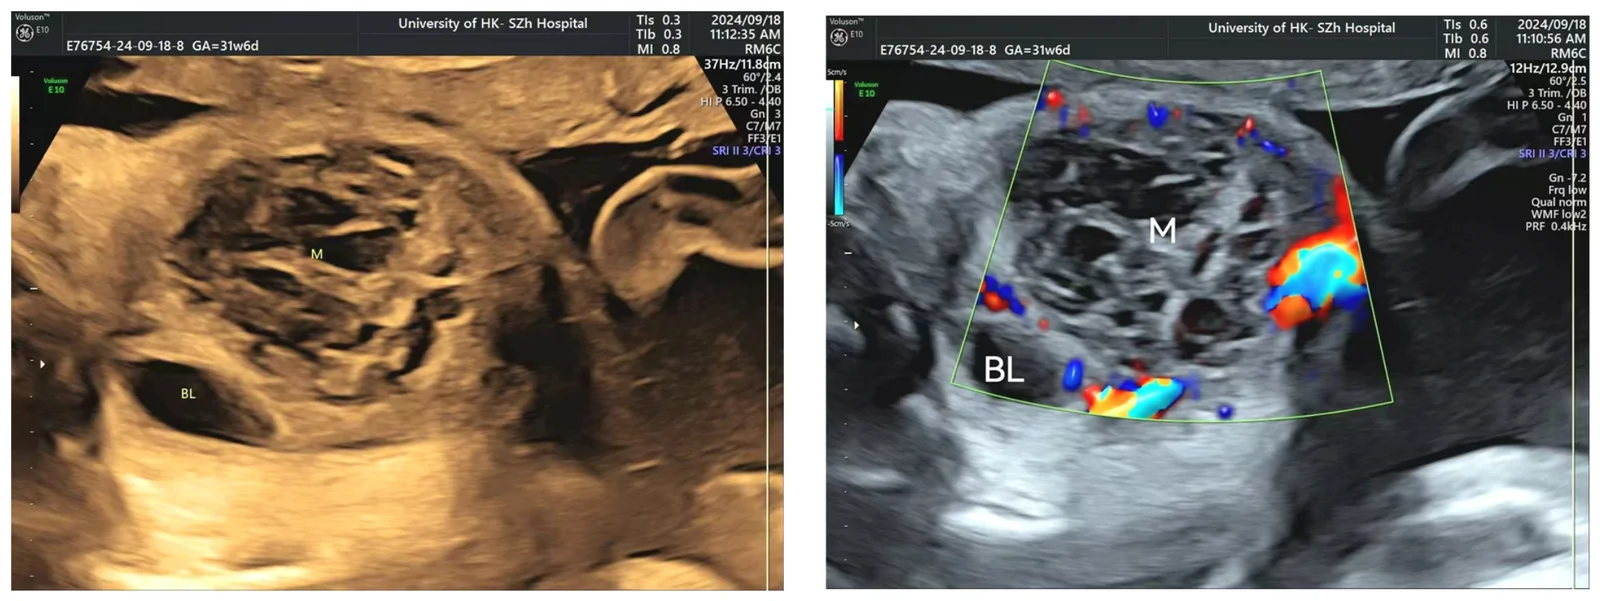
Fetal ultrasound (31 weeks): A mixed mass (postoperatively confirmed as a collapsed colon) is shown at the bottom of the pelvic cavity (left image). CDFI reveals point-line-like blood flow signals around the mass (right image). BL: bladder; M: mass.
